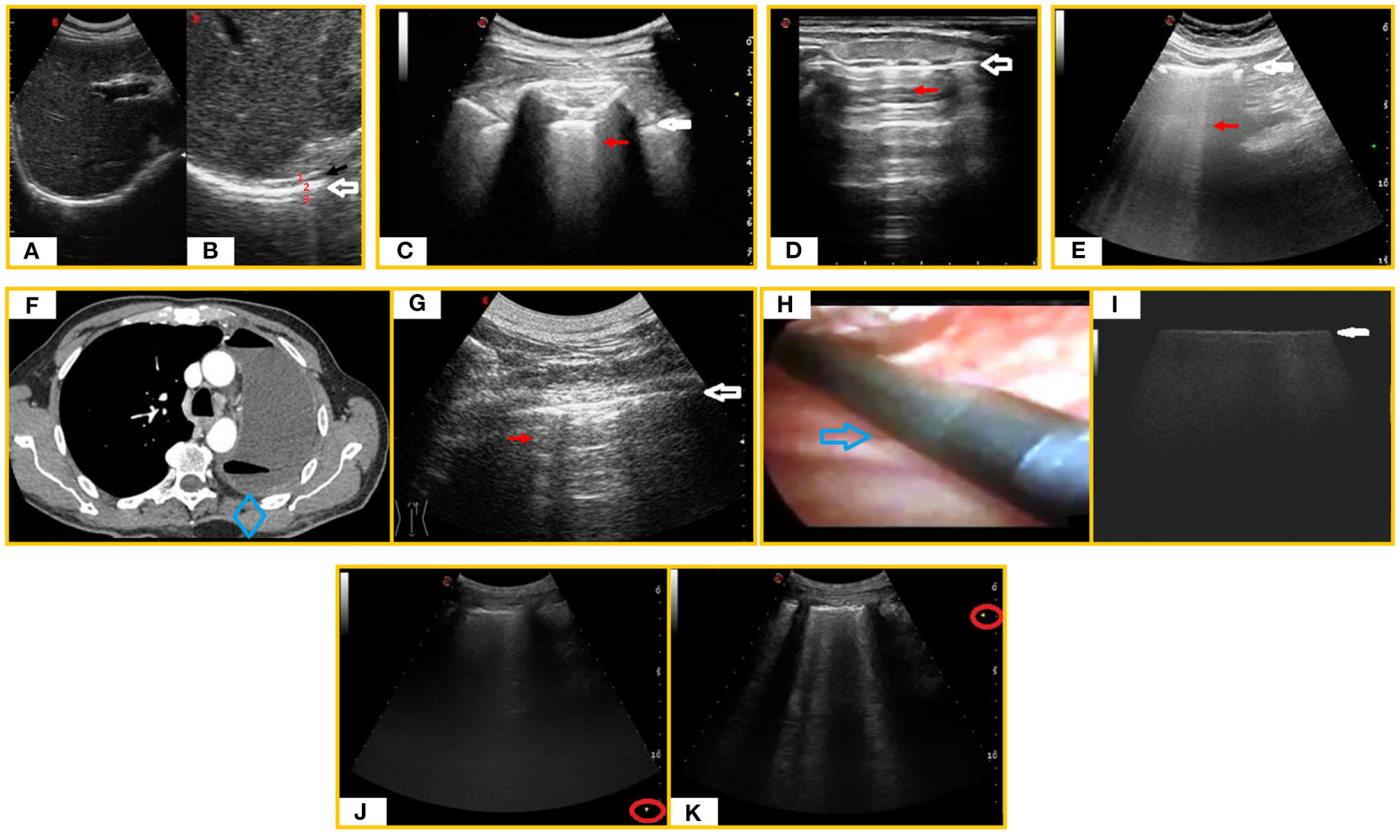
LUS artifacts. (A) Subcostal right ultrasound (US) scan with a convex probe (6 MHz). (B) Magnification on the “diaphragmatic hyperechoic line” (white arrow) showing that it is actually composed of three hyperechoic lines (1. hepatic capsule/diaphragmatic muscle interface line, 2. diaphragmatic muscle/pulmonary base interface line, 3. mirror reflection artifact) within which the real diaphragmatic muscle appears as a thin hypoechoic line (black arrow). (C) LUS scan with a convex probe (6 MHz) in a healthy individual showing the “hyperechoic pleural line” (white arrow) followed by a sporadic B-line (red arrow). (D) Longitudinal tracheal US scan with a linear probe (8 MHz) showing a “tracheal hyperechoic line” at the soft tissue/tracheal air interface (white arrow) followed by reverberation artifacts (red arrow). (E) Abdominal US scan with a convex probe (6 MHz) showing a “hyperechoic peritoneal line” (white arrow) followed by laser-like vertical and confluent ring down artifacts (red arrow). (F) Axial chest CT scan in a post-pneumonectomy patient revealing air and fluid collection in the residual space with mediastinal sliding. The intact lung is shifted toward the residual cavity. (G) US scan of the post-pneumonectomy space [corresponding to the blue box in the (F) CT scan] revealing a hyperechoic interface line (white arrow) followed by reverberation artifacts (red arrow). (H) Image of the pulmonary parenchyma of a patient diagnosed with usual interstitial pneumonia UIP during VATS. A sterile intracavitary laparoscope probe (12 MHz) with 10-mm diameter and 38-cm length (blue arrow) was introduced through one of the VATS ports in the thoracic cavity. (I) Intraoperatory LUS scan (linear probe, 12 MHz) showing an irregular increased thickness of the pleura line (white arrow) with no artifact below it. (J,K) LUS scans showing how modification of focus position (red round) can modify the number of vertical artifacts.
